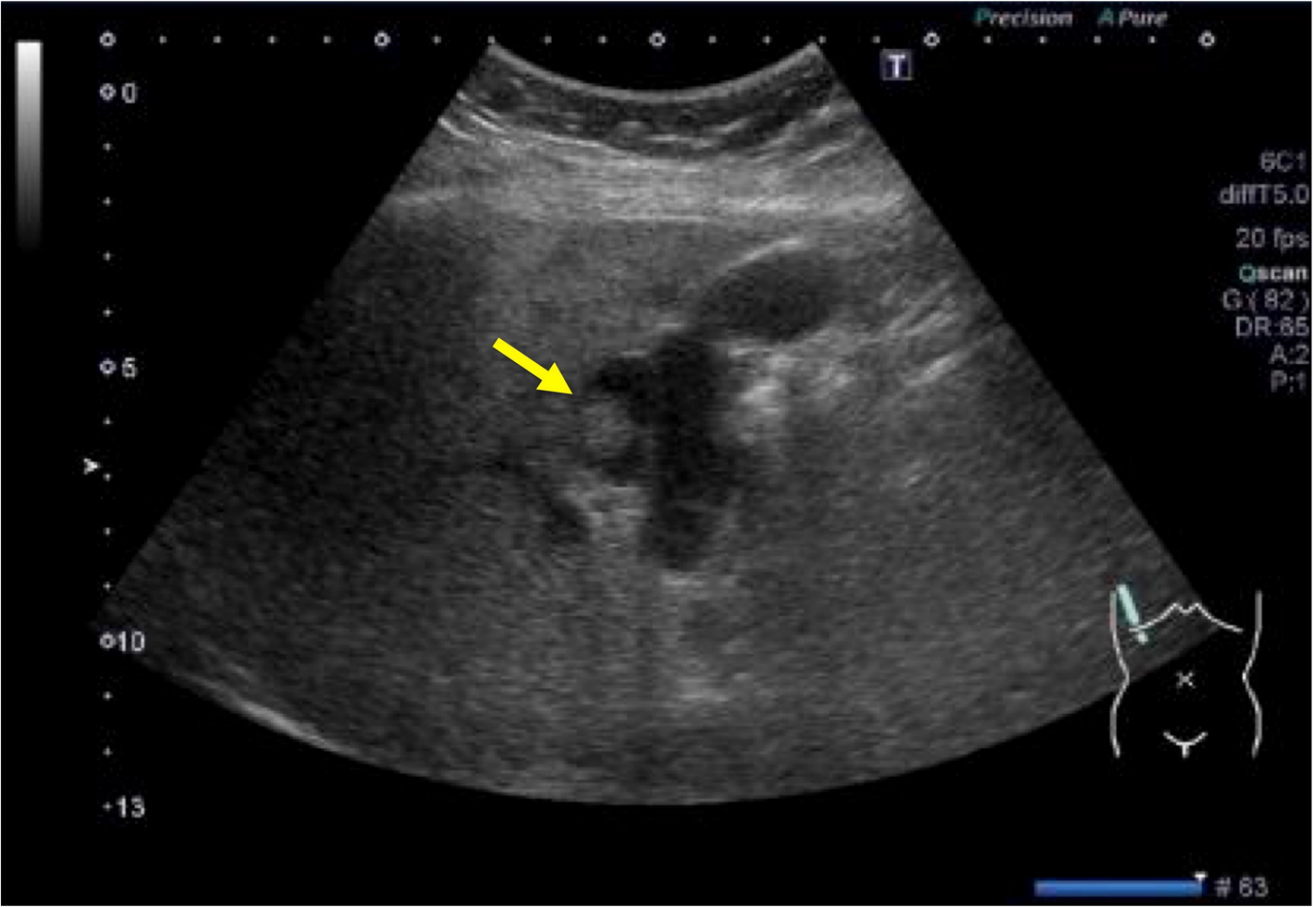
Ultrasonography imaging. The arrow shows carcinoma 1 cm in diameter in the proximal gallbladder with a base and obscure demarcation along the wall
